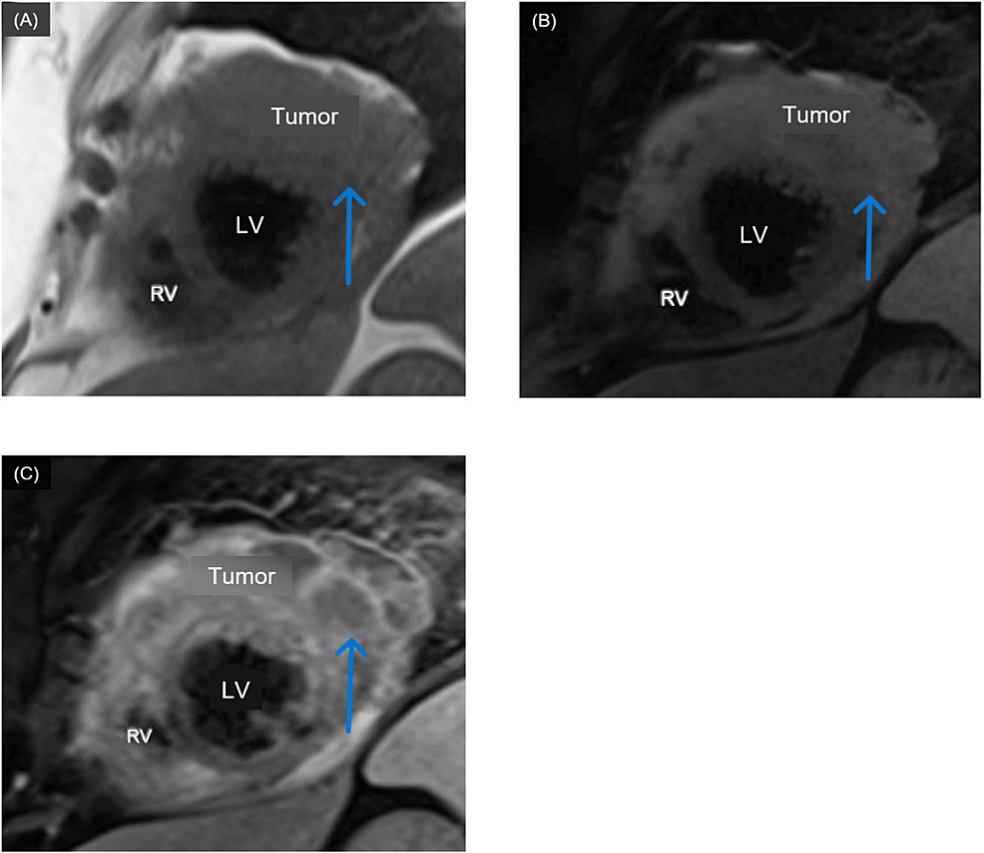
CMRI. Apical pre-gadolinium T1W slice (A), apical pre-gadolinium T2W slice (B), and apical post-gadolinium T1W slice (C). The tumor (blue arrows) is most obvious on the post-gadolinium enhancement image. LV: Left ventricle, RV: Right ventricle; CMRI: Cardiac MRI
